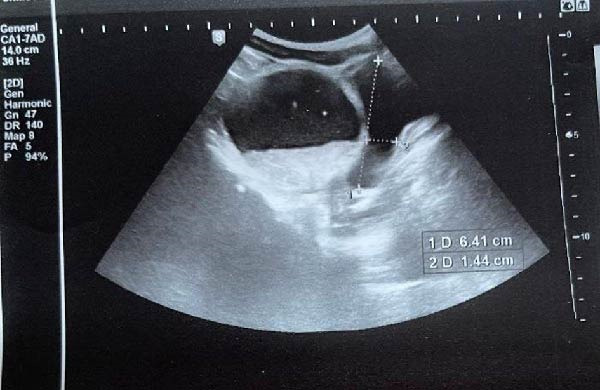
Abdominal ultrasonography demonstrating free intraperitoneal fluid and a large para‐vesical cystic mass measuring 12.5 cm × 7 cm.
